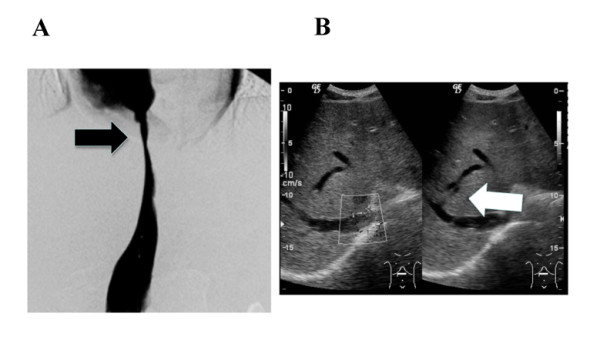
Images showing various features of re-stenosis after the first PTA. One year after the first PTA, angiograms of the IVC showed severe stricture of the IVC at the level of the hepatic veins (A: black arrow). Doppler US showed that the RHV and MHV were completely occluded with regurgitation in MHV, and thick shunt vessels from MHV to RHV were noticed (B: white arrow).
